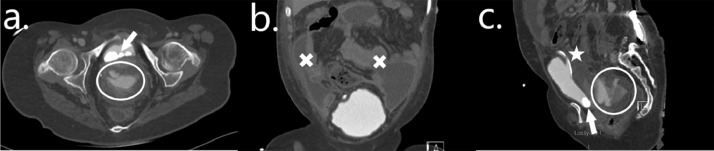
Case 3—Spontaneous intraperitoneal bladder perforation: Axial (A), coronal (B), and sagittal (C) images of a CT urogram. Active contrast extravasation can be seen (circles) with a large volume of intraperitoneal fluid (crosses). Bladder stones (arrows) can be seen within the augmented bladder with a suprapubic catheter (star) in situ.
